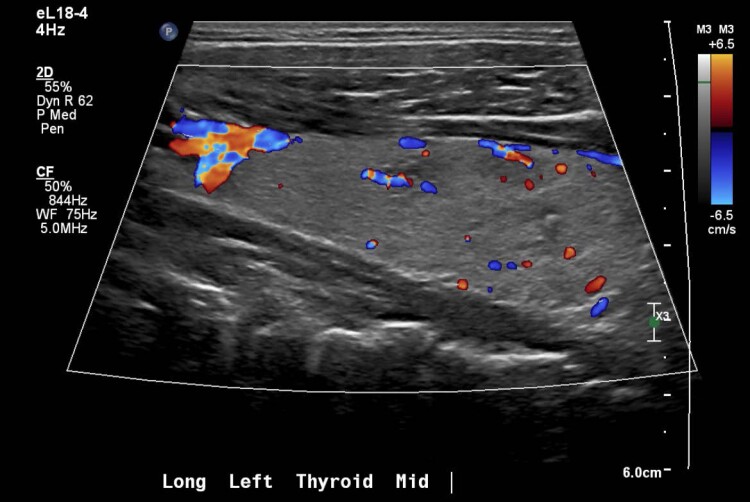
Longitudinal: left medial mid gland with Doppler.
